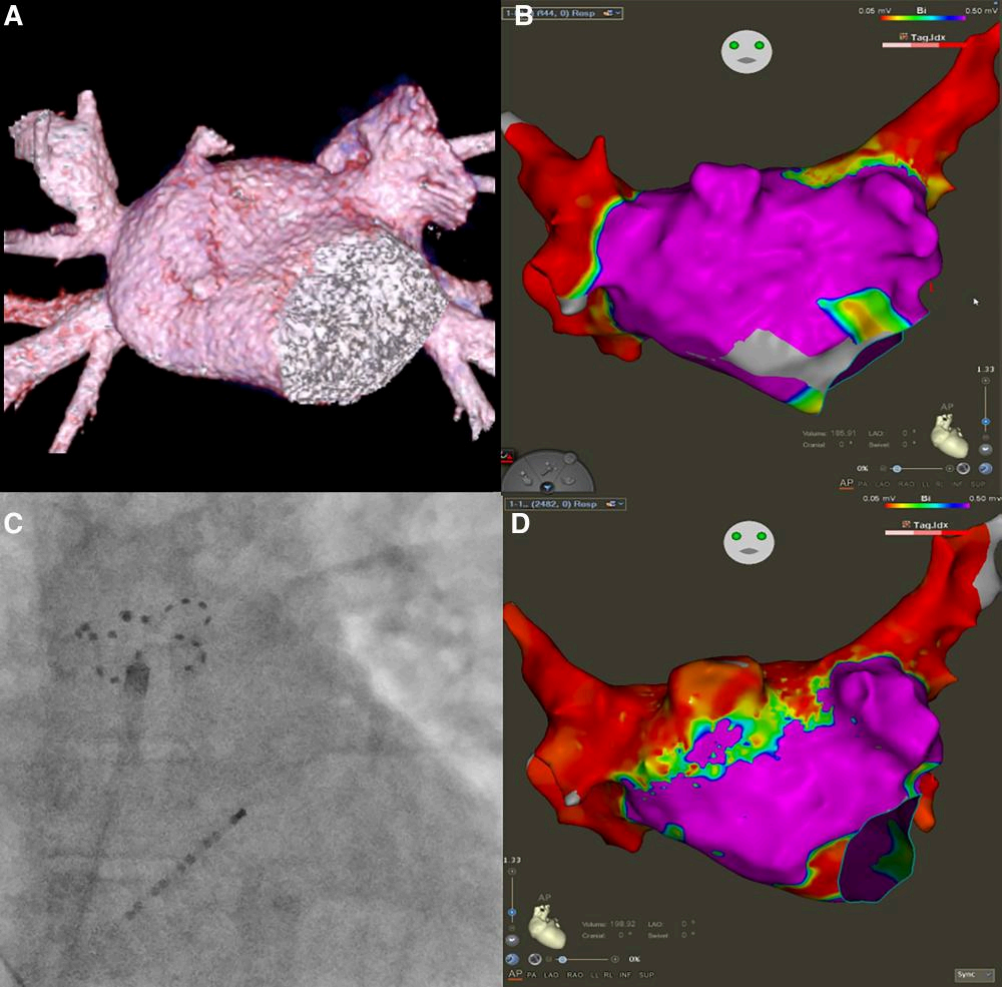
Under deep conscious sedation, a single transseptal puncture was performed. 3D electroanatomical mapping (3D-EAM) showed durable isolation of all PVs and no evidence of extra-PV low-voltage scar. An outpouching consistent with an accessory left atrial (LA) appendage (aLAA) was found on the LA anterior-superior aspect anterior to right superior pulmonary vein (RSPV) with large electrical signals and confirmed after review of a prior cardiac computed tomography scan (*Panels A* and *B*).
